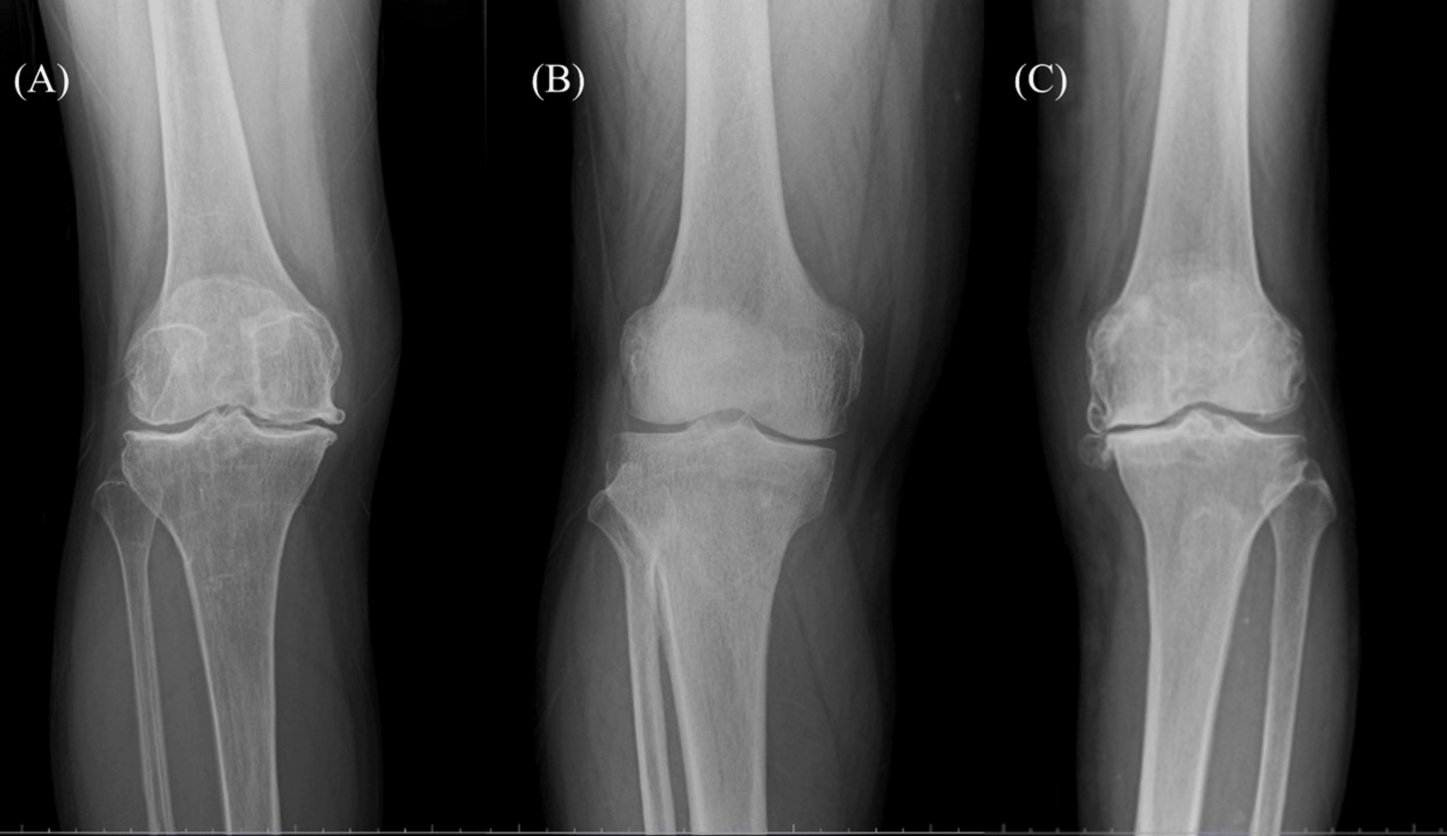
Frontal knee radiographs of the three cases demonstrating Kellgren-Lawrence grade 3 knee osteoarthritis (A) Case 1, (B) Case 2, and (C) Case 3
